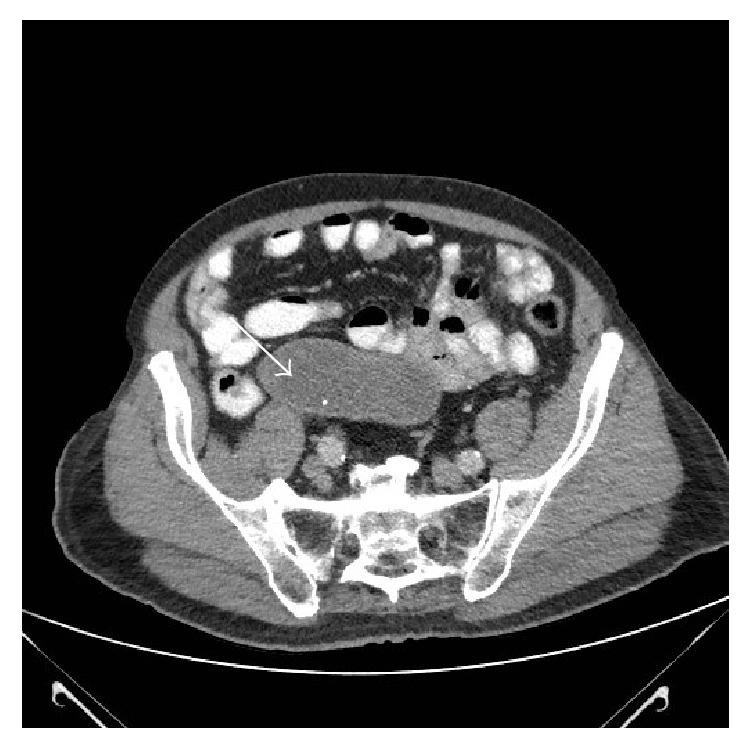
Follow-up CT abdomen pelvis showing a dilated tubular structure arising from the medial aspect of the caecum with unchanged features suggestive of a benign appendicular mucocele and no other intra-abdominal pathology.
